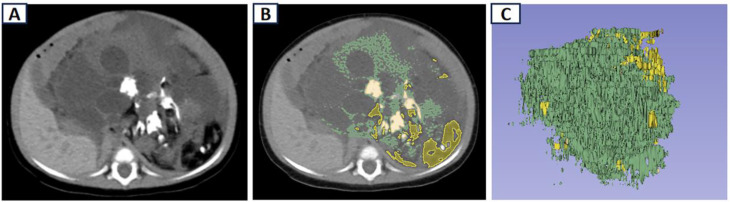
Delineation of teratoma VOI on CT. (A) CT; (B) semi-automatic delineation of VOIs of fat, calcification and solid component; (C) the VOI obtained. VOI, volume of interest; CT, Computed tomography; VOI, volume of interest
